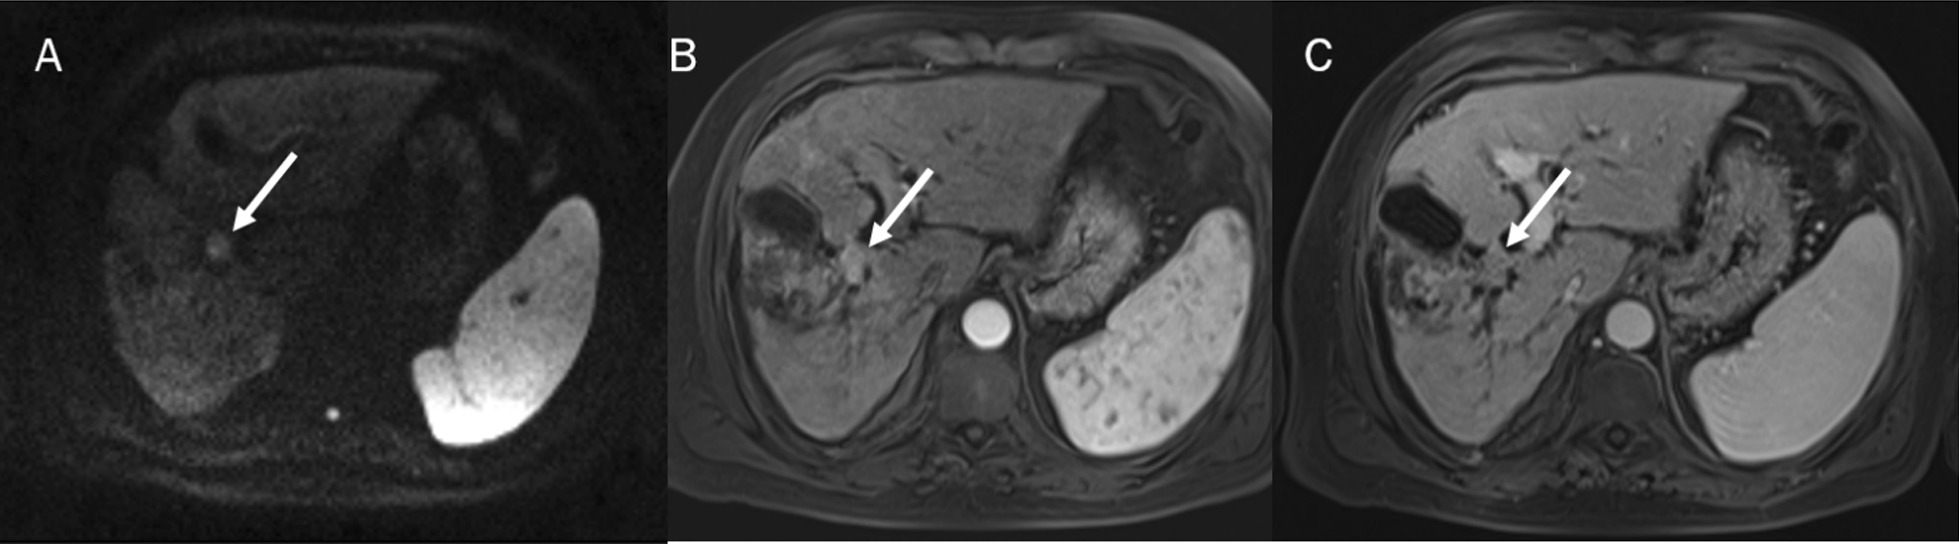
In DWI (A: b 800 s/mm2)) the lesion shows restricted signale and arterial hyperenhancement (B) with progressive enhancement (C) during contrast stuy
